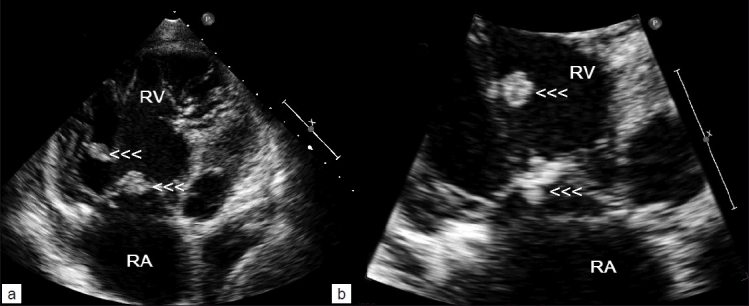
Transthoracic echocardiography (a and b) showing multiple vegetations attached to tricuspid valve leaflets (arrowheads) and one large vegetation on the chordae of anterior tricuspid leaflet (upper arrowheads) in a patient with IV drug abuse and septic pulmonary emboli. RA, right atrium; RV, right ventricle
